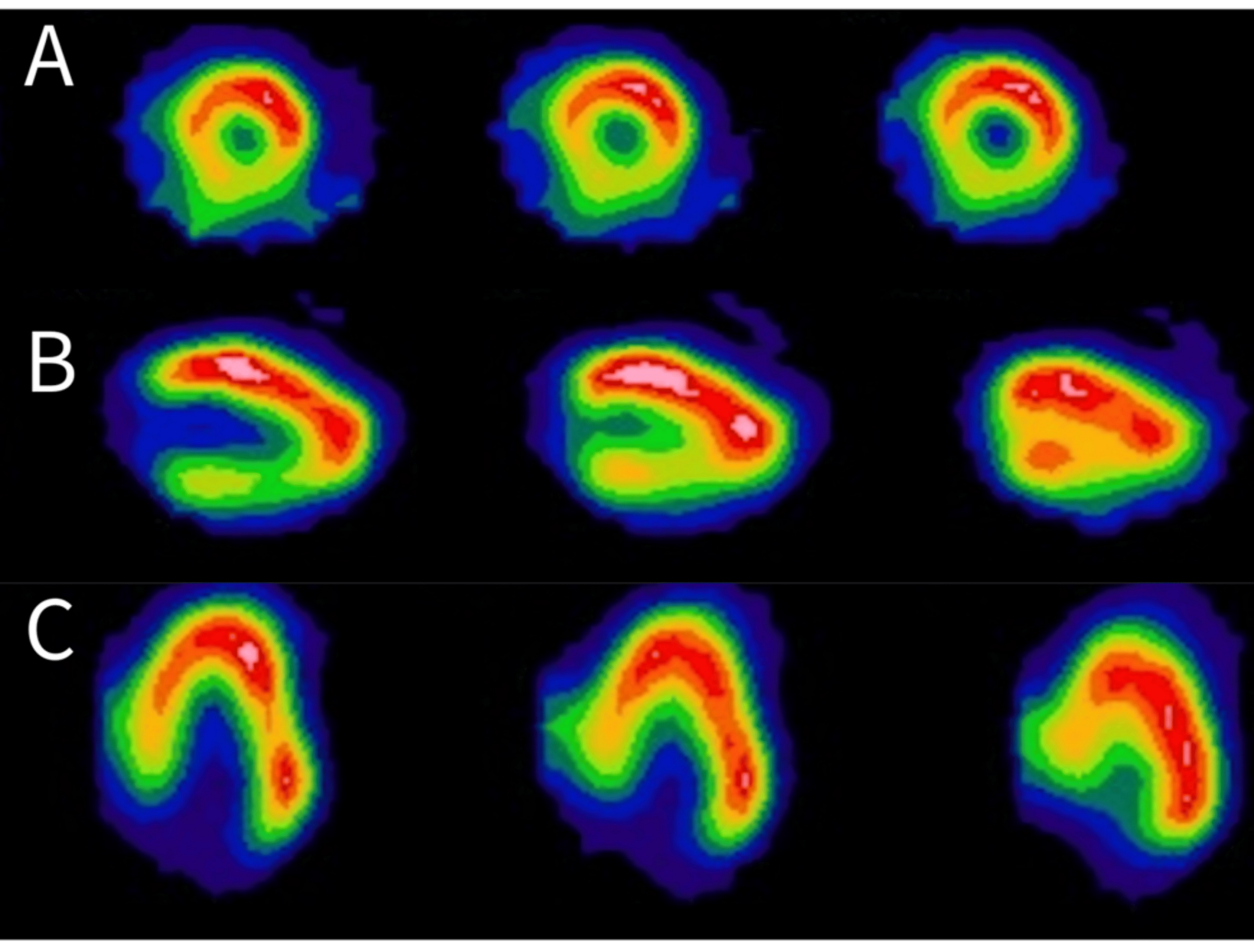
Positive Tc-99 m PYP scan demonstrating increased myocardial tracer uptake. Cardiac SPECT showing diffuse myocardial tracer uptake in short-axis images (A, top row), horizontal long-axis (B, middle row), and vertical-long axis images (C, last row). This scan is diagnostic of ATTR cardiomyopathy. Tc-99 m PYP: Technetium-99 m pyrophosphate; SPECT: single photon emission computed tomography; ATTR: transthyretin amyloid
